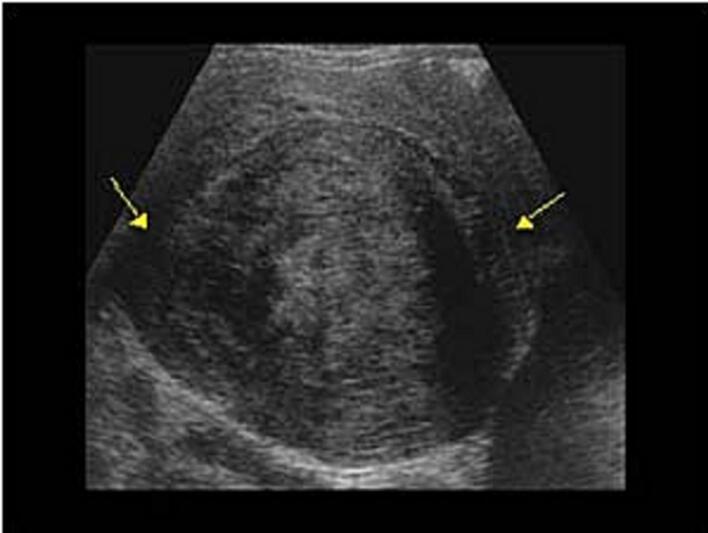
Abdominal ultrasound showing large subserosa fibroid on the dorsal aspect of the uterus.
